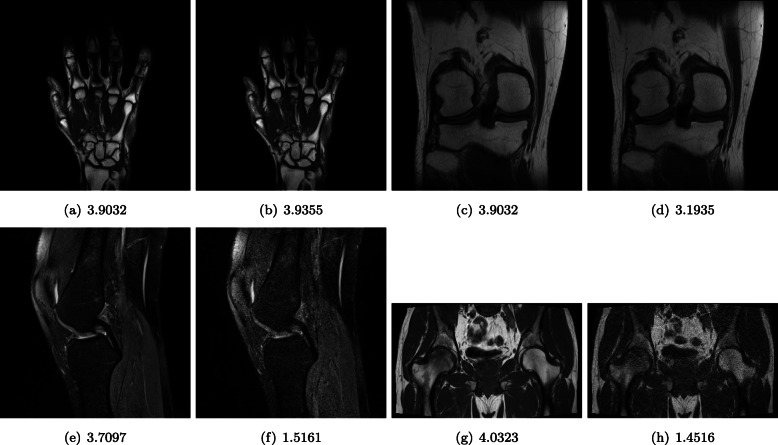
T2-weighted images and their mean opinion scores. The images of the wrist and knee are of the same quality (cf. (a) and (c)), despite differences in the structure of the tissue and bones. The appearance of structures was perceived worse for the degraded knee (e) than shown in (d) due to the plane of the acquisition. Severely degraded images of different body parts were assessed similarly (f,h)
